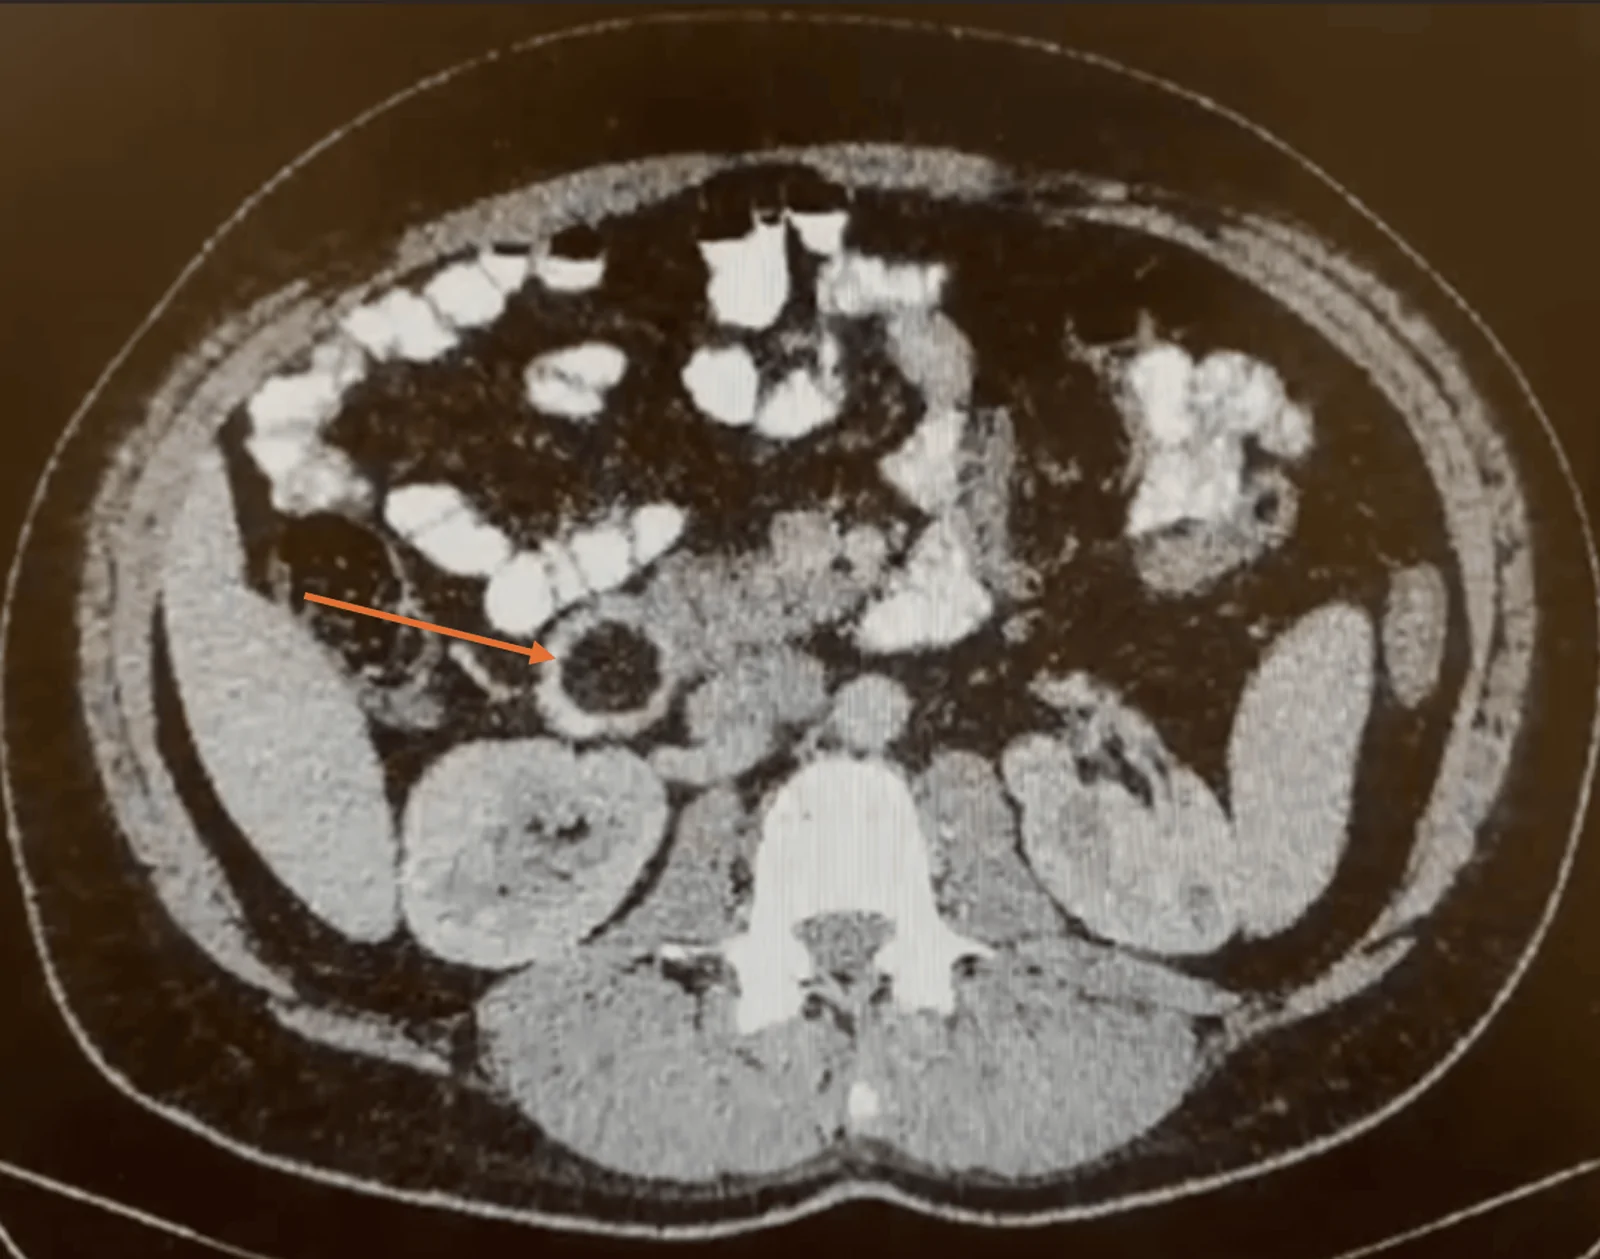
CT showing a solitary, well-defined, fat-density mass in the duodenum causing partial luminal obstruction CT: computed tomography
